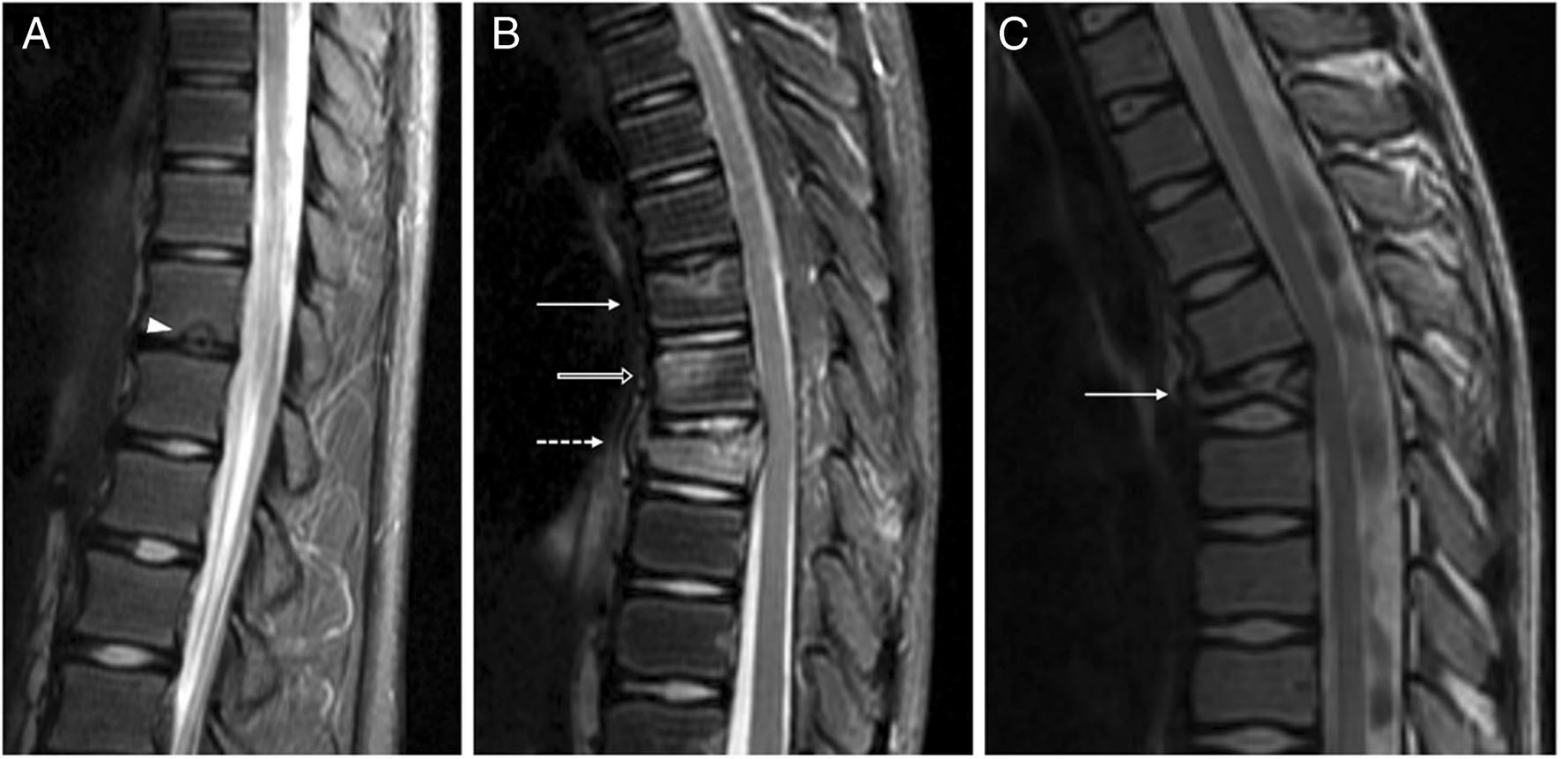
Schmorl node and platyspondyly with different degrees of vertebral height loss in three different patients with chronic nonbacterial osteomyelitis: magnetic resonance images of the dorsal spine obtained from a 14-year-old boy (a), a 12-year-old boy (b), and a 12-year-old girl (c). a Sagittal T2-weighted fat-saturated image shows a Schmorl node in the inferior endplate of dorsal (D) vertebra 11 (*arrowhead*). b Sagittal T2-weighted fat-saturated image shows platyspondyly of D6 with less than 50% of vertebral body height loss (*arrow*), bone edema of the body of D7 (*open arrow*) without vertebral height loss, and platyspondyly of D8 with more than 50% of vertebral body height loss (*broken arrow*). Note also the bone edema adjacent to the superior endplate in D6, and the pre- and post-somatic soft tissue inflammation around D8. c Sagittal T2-weighted image shows severe vertebral height loss of D6, with a vertebra plana appearance (*arrow*)
